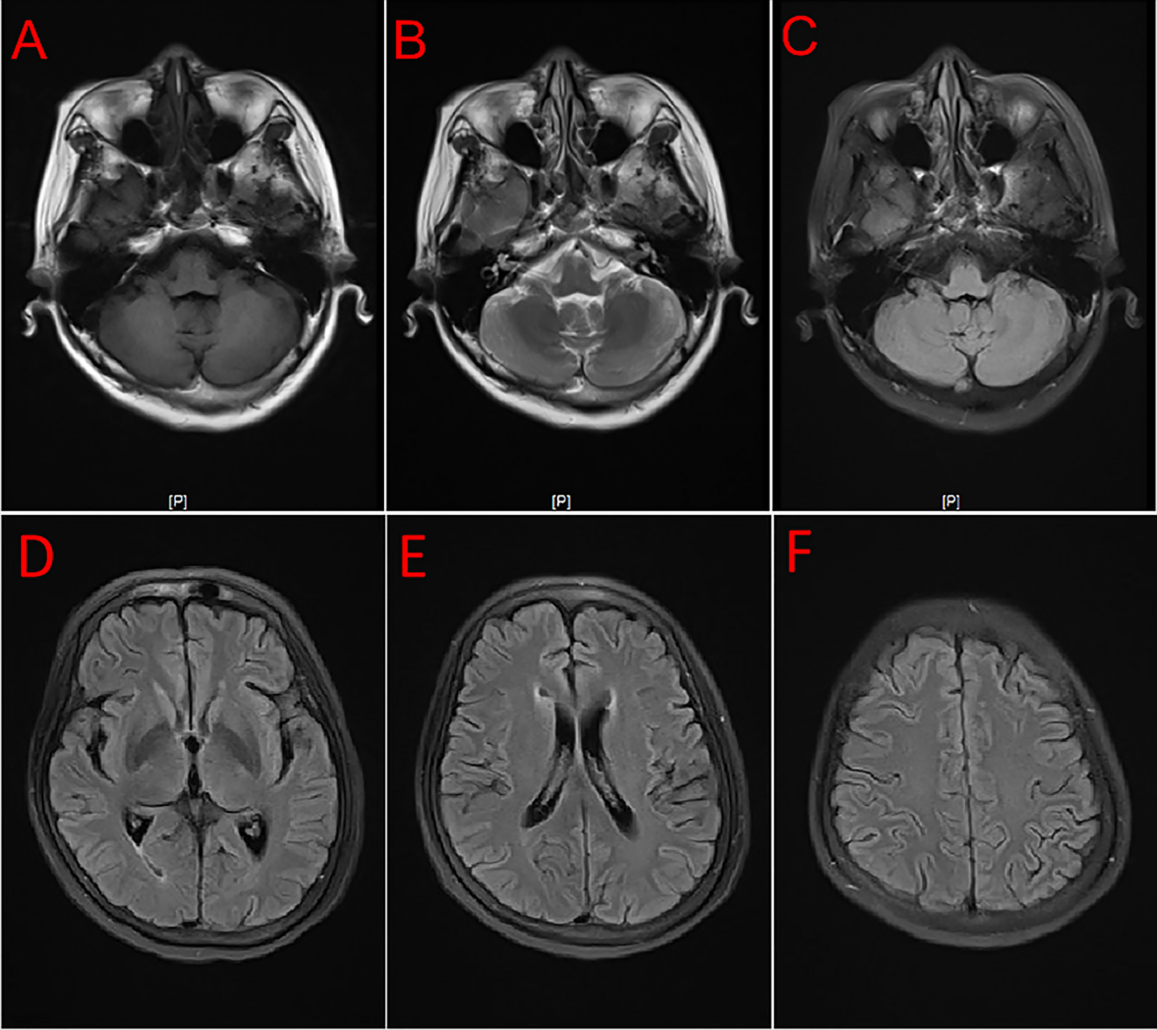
MRI showed no typical imaging features for APS and brain lesions. T1 (A), T2 (B), and Flair (C–F).
